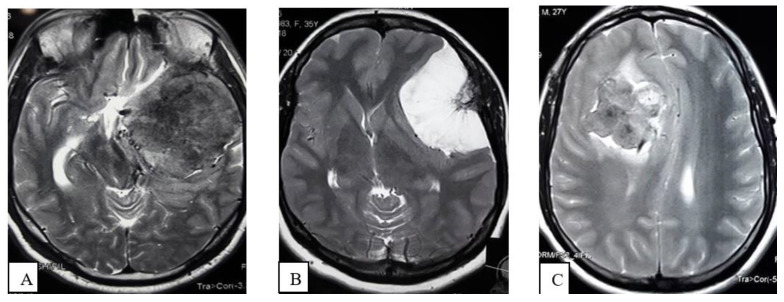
MRI of Brain, T2WI axial section demonstrates Iso- to hypointense (A), Hyperintense (B), Mixed intensity (C) tumor.
